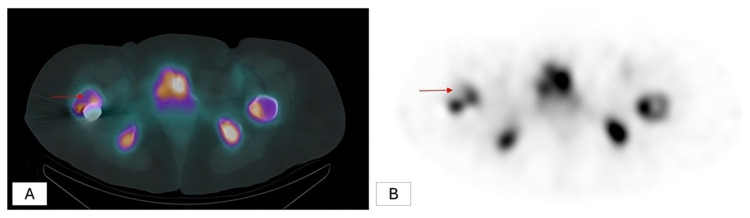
The distinction between viable and nonviable was made based on radionuclide uptake on SPECT/CT images (anterior view [A] and posterior view [B]).
